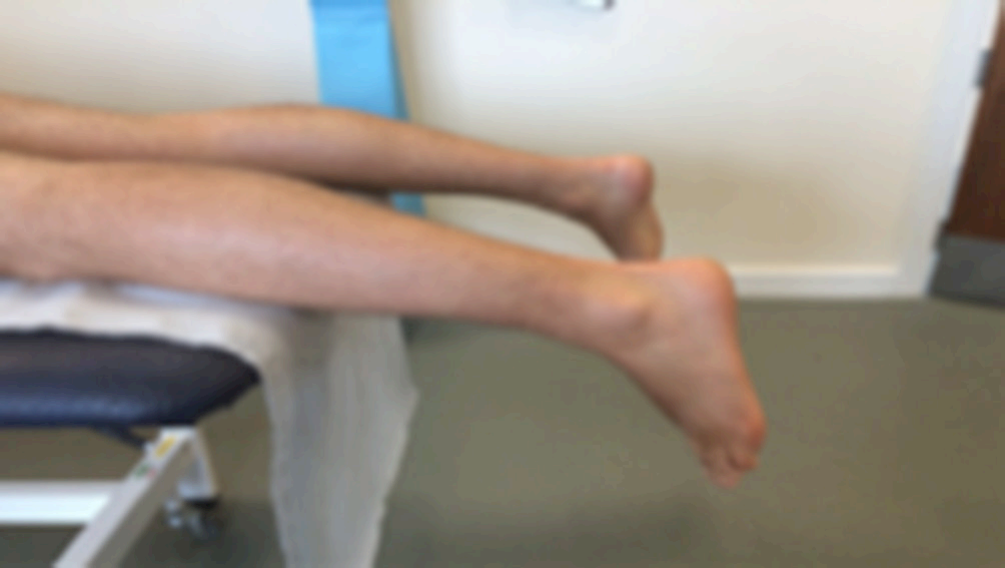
Standardised position for ultrasound imaging of the Achilles tendon.
